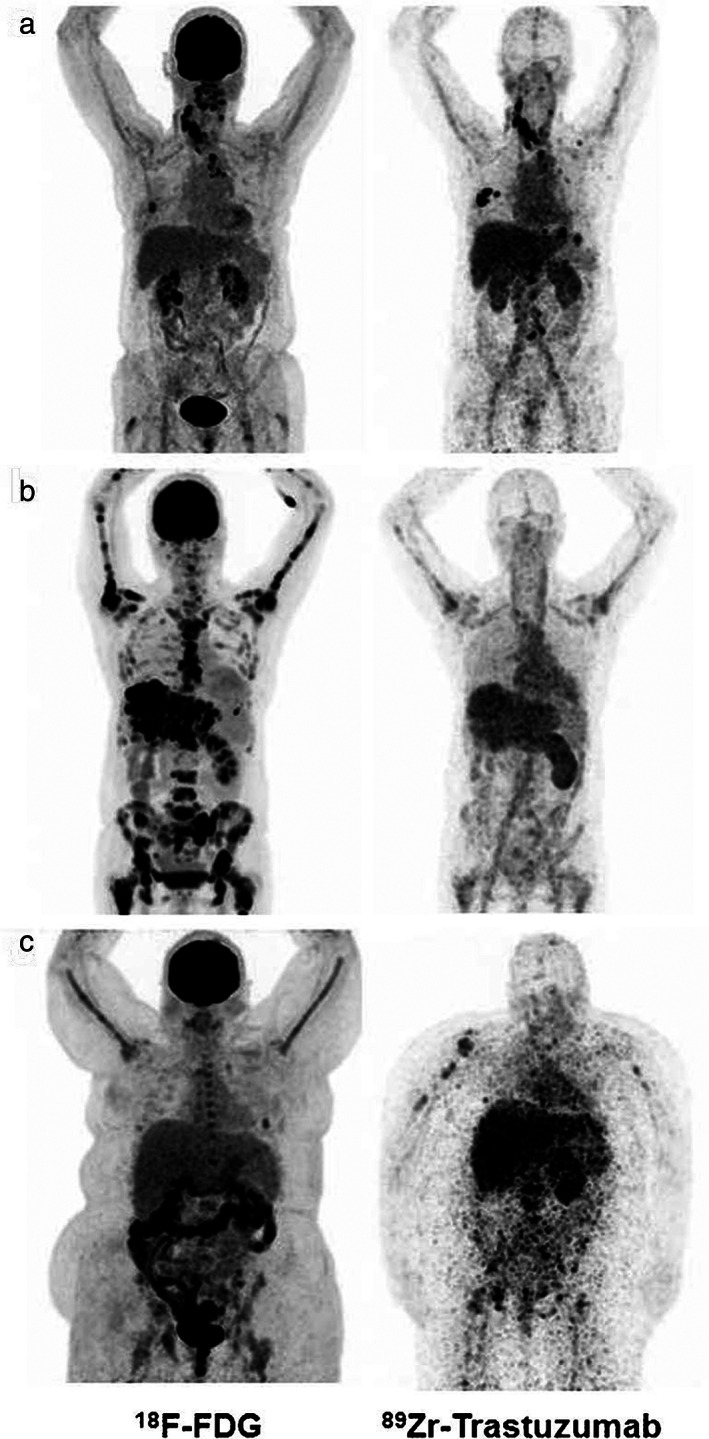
^18^F‐FDG (*left*) and ^89^Zr‐trastuzumab PET scans (*right*) of three patients: Example of a patient with a ^89^Zr‐trastuzumab PET scan considered HER2‐positive (a), a ^89^Zr‐trastuzumab PET scan considered HER2‐negative (b), and an ^89^Zr‐trastuzumab PET scan considered equivocal (c). Reprinted under a Creative Commons (CC BY 4.0) license from: Bensch F, Brouwers AH, Lub‐de Hooge MN, de Jong JR, van der Vegt B, Sleijfer S, de Vries EGE, Schröder CP. ^89^Zr‐trastuzumab PET supports clinical decision making in breast cancer patients, when HER2 status cannot be determined by standard work up. Eur J Nucl Med Mol Imaging. 2018;45(13):2300–2306.
